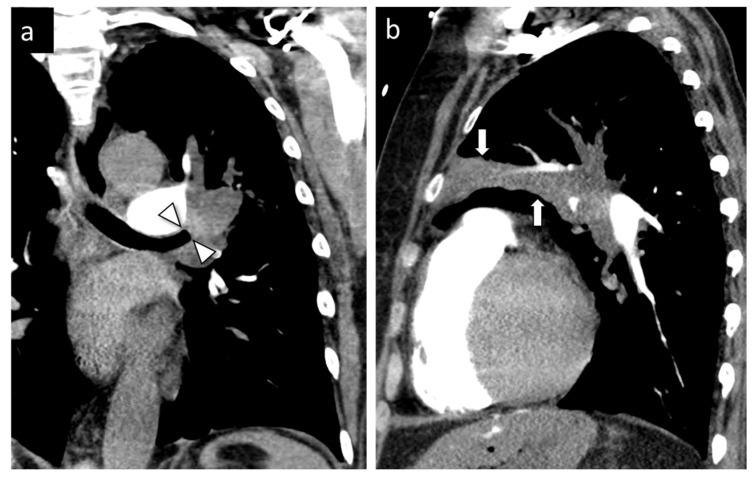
(a,b): CT coronal (a) and sagittal images (b) of a 59-year-old woman with left perihilar primary squamous cell lung carcinoma obstructing the left upper lobar bronchus (arrowheads), yielding segmental post obstructive atelectasis (arrows).
